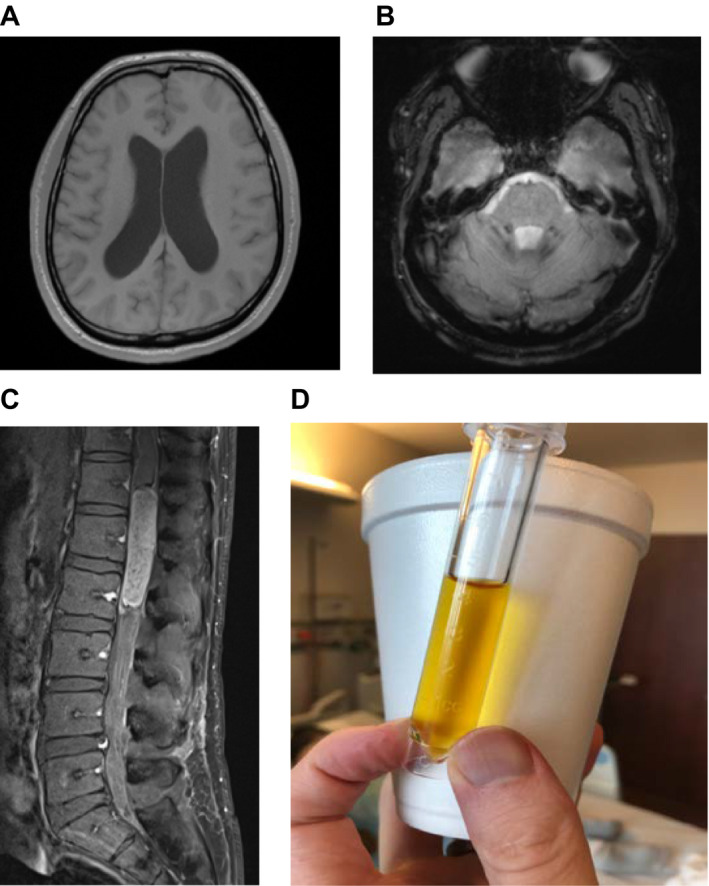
A. T1 MRI Axial of brain demonstrating hydrocephalus B. GRE MRI Axial of brain demonstrating hemosiderin deposits in the bilateral cerebellar hemispheres concerning subarachnoid hemorrhage C. Intramedullary mass demonstrated on sagittal MRI lumbar spine D. Cerebrospinal fluid from lumbar puncture demonstrating xanthrochromia.
